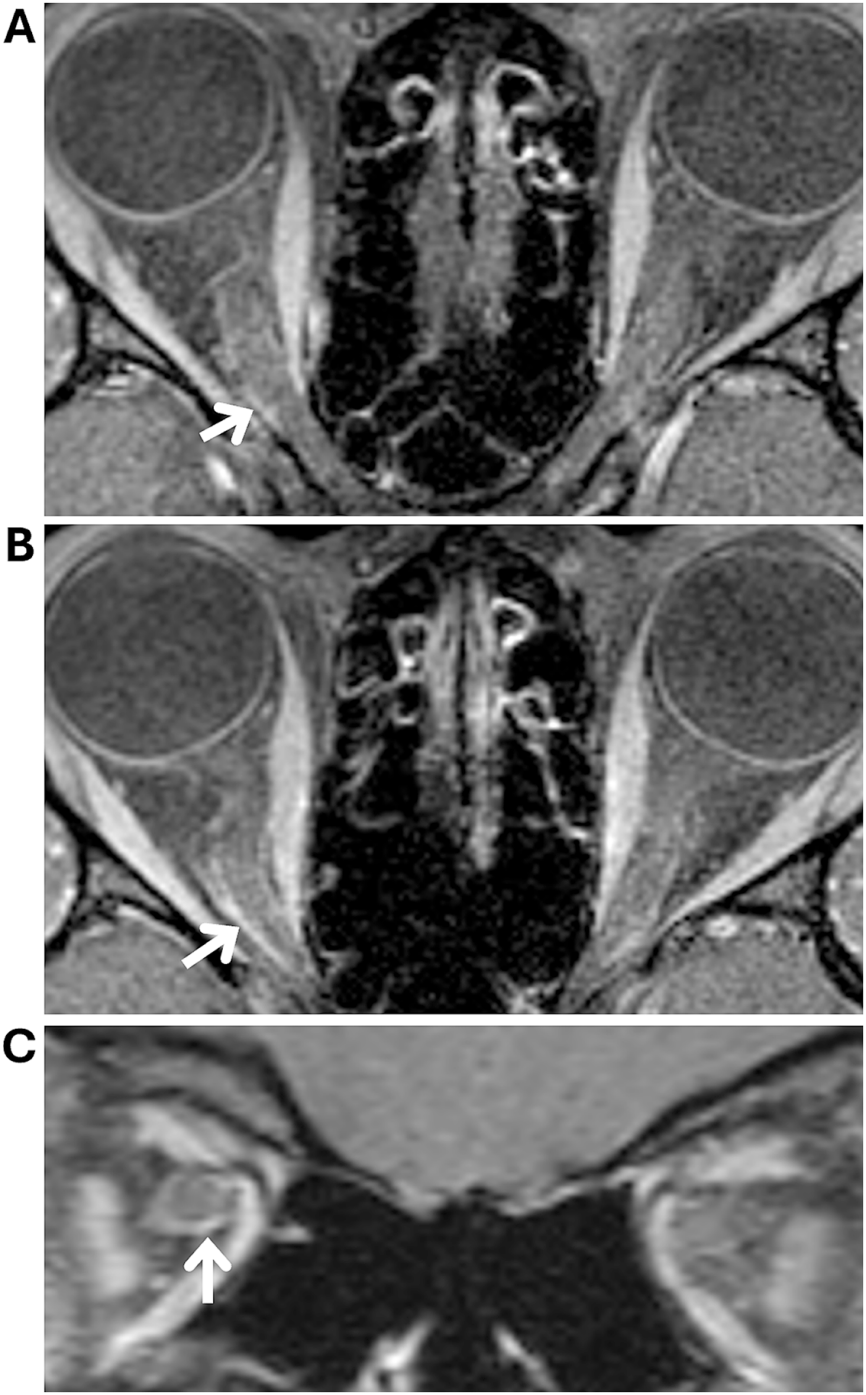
Magnetic resonance imaging (MRI) of a patient with a new diagnosis of idiopathic intracranial hypertension, categorized as having suggested optic nerve sheath enhancement versus blood vessels (patient two in Table 1). Fat-suppressed T1-weighted orbital MRI with contrast (A–C) in axial (A,B) and coronal (C) planes showing areas suggestive of optic nerve sheath enhancement (white arrows).
